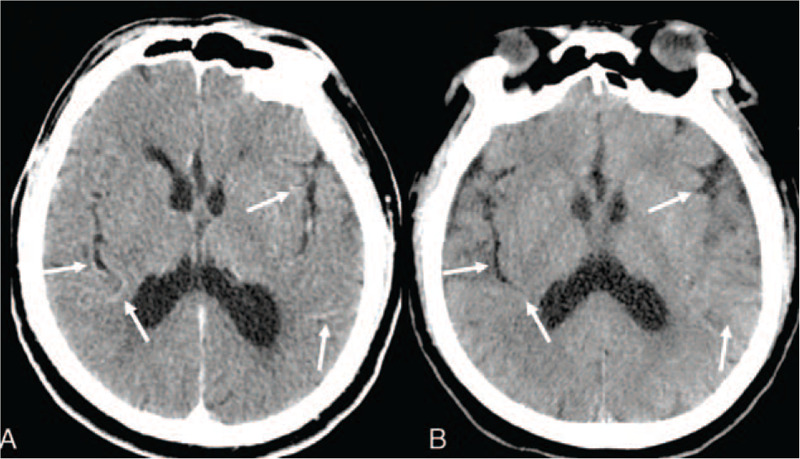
Plain brain CT acquired immediately and 2 days after admission A, Plain brain CT images showed abnormal cortical contrast enhancement and cerebral sulci hyperdensity. B, Plain brain CT images acquired 2 days after admission showed no abnormalities.
